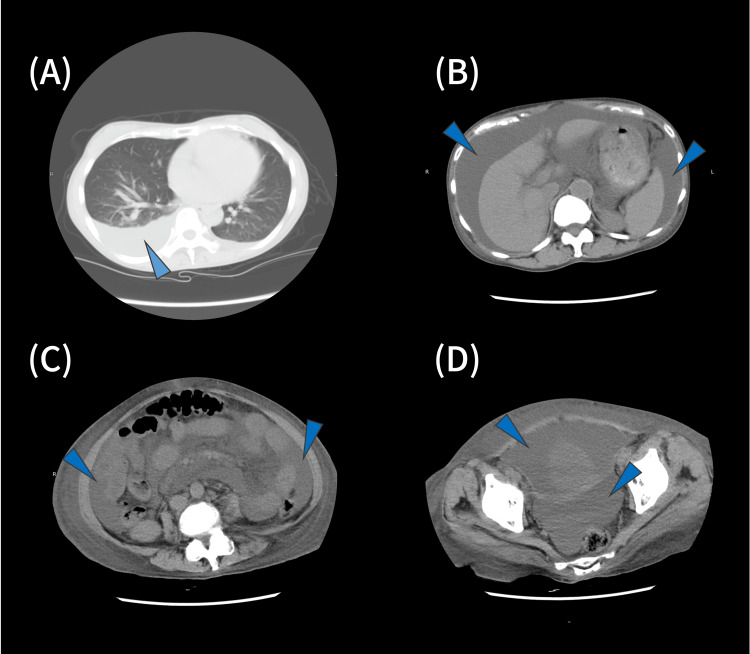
A non-enhanced computed tomography (chest to pelvis) (A) chest imaging; (B) abdominal imaging (upper); (C) abdominal imaging (middle); (D) abdominal imaging (lower). Right pleural effusion and ascites (blue arrowhead) were observed, but no obvious malignancy was detected.
